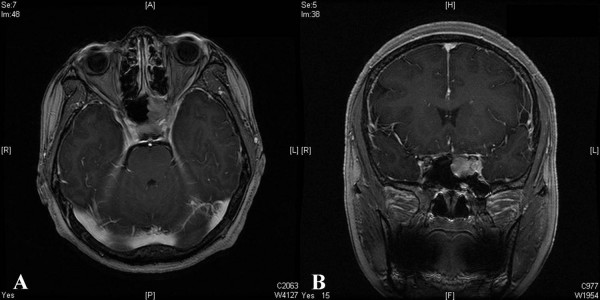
Axial (A) and sagittal (B) preoperative Gadolinium-enhanced MRI, demonstrating a homogenous soft-tissue lesion occupying the left sphenoid sinus and invading the left cavernous sinus.
